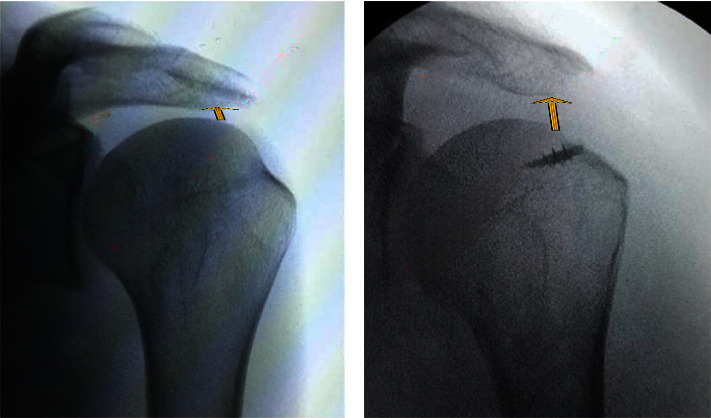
(a) Preoperative and 1-year postoperative (b) X-ray images of a patient's shoulder undergoing open surgery. The postoperative image shows increased subacromial space following acromioplasty. The patient reported significant pain relief and improved range of motion at 1 year of follow-up.
